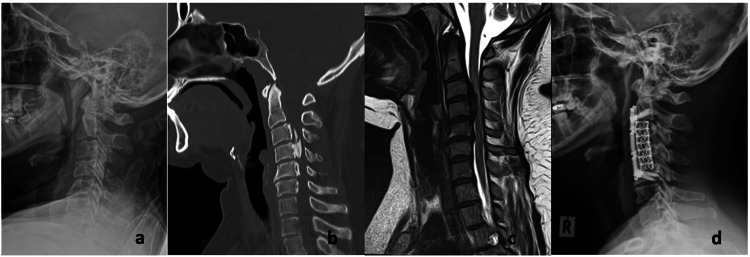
Representative case of a patient who has undergone anterior cervical corpectomy and fusion a: Plain radiograph showing neutral cervical lordosis; b: CT imaging with segmental OPLL involved from C3 to C5 and occupying ratio of more than 60%; c: MRI image showing spinal cord compression without hyperintensity of the spinal cord; d: Anterior cervical corpectomy of C3 and C4 OPLL: Ossification of the posterior longitudinal ligament
